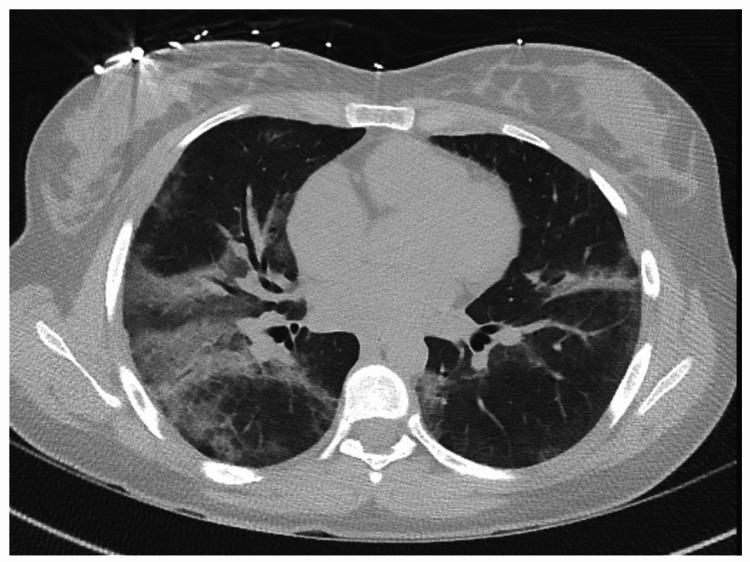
Chest CT of case 3 remarkable for bilateral patchy GGOs. CT: computed tomography; GGOs: ground-glass opacities
